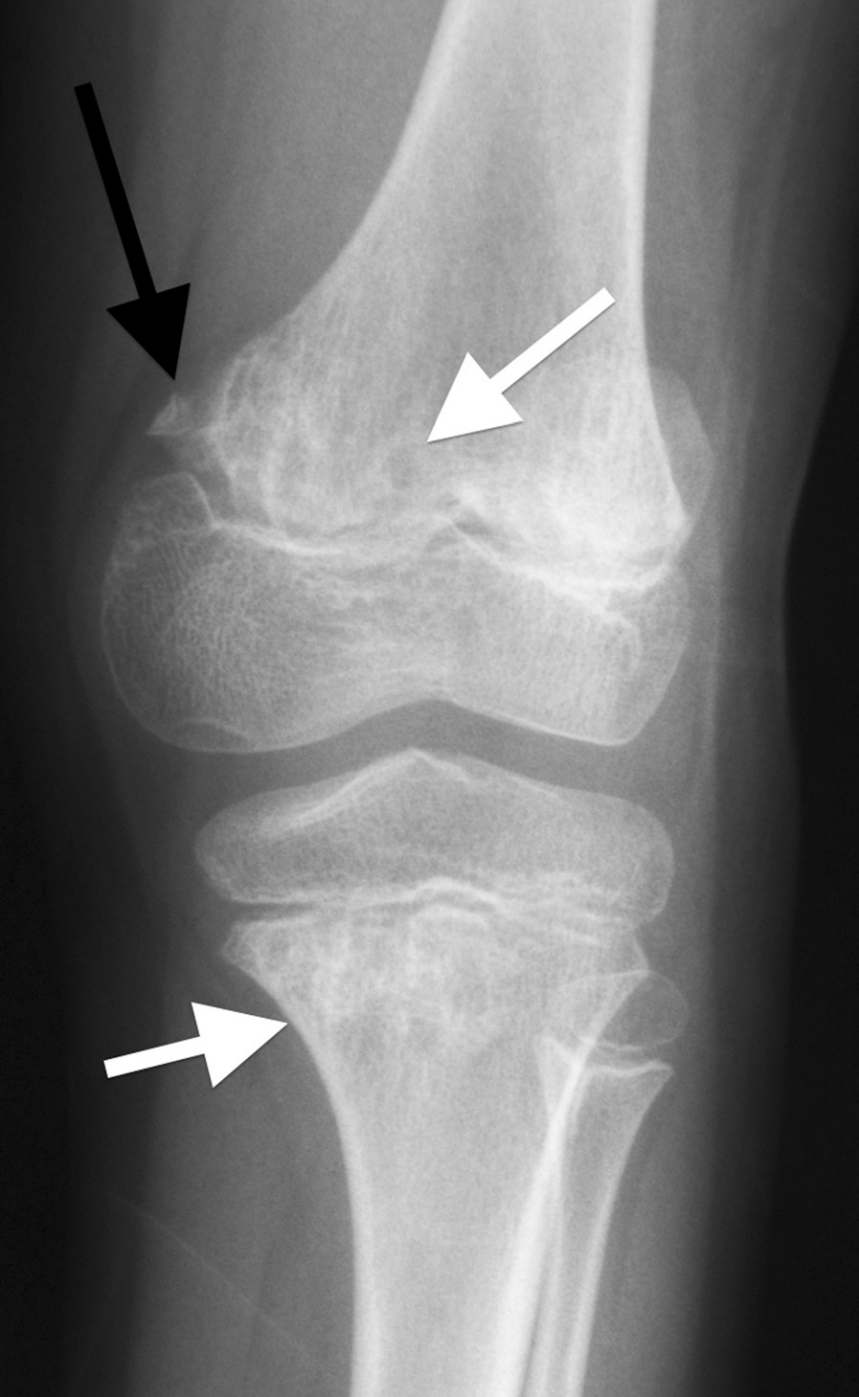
Knee radiograph of UPN1. There is sclerosis and lucency in the distal femoral and proximal tibial metaphyses (white arrows) with pseudofragmentation of the medial part of the distal femoral metaphysis (black arrow).
